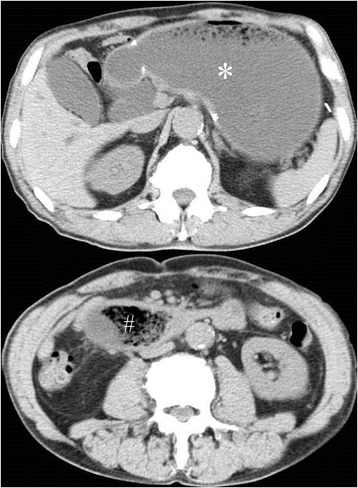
Computed tomography (CT) on admission. CT revealed dilatation of the remnant stomach (*) and a bezoar in the third portion of the duodenum (#)
